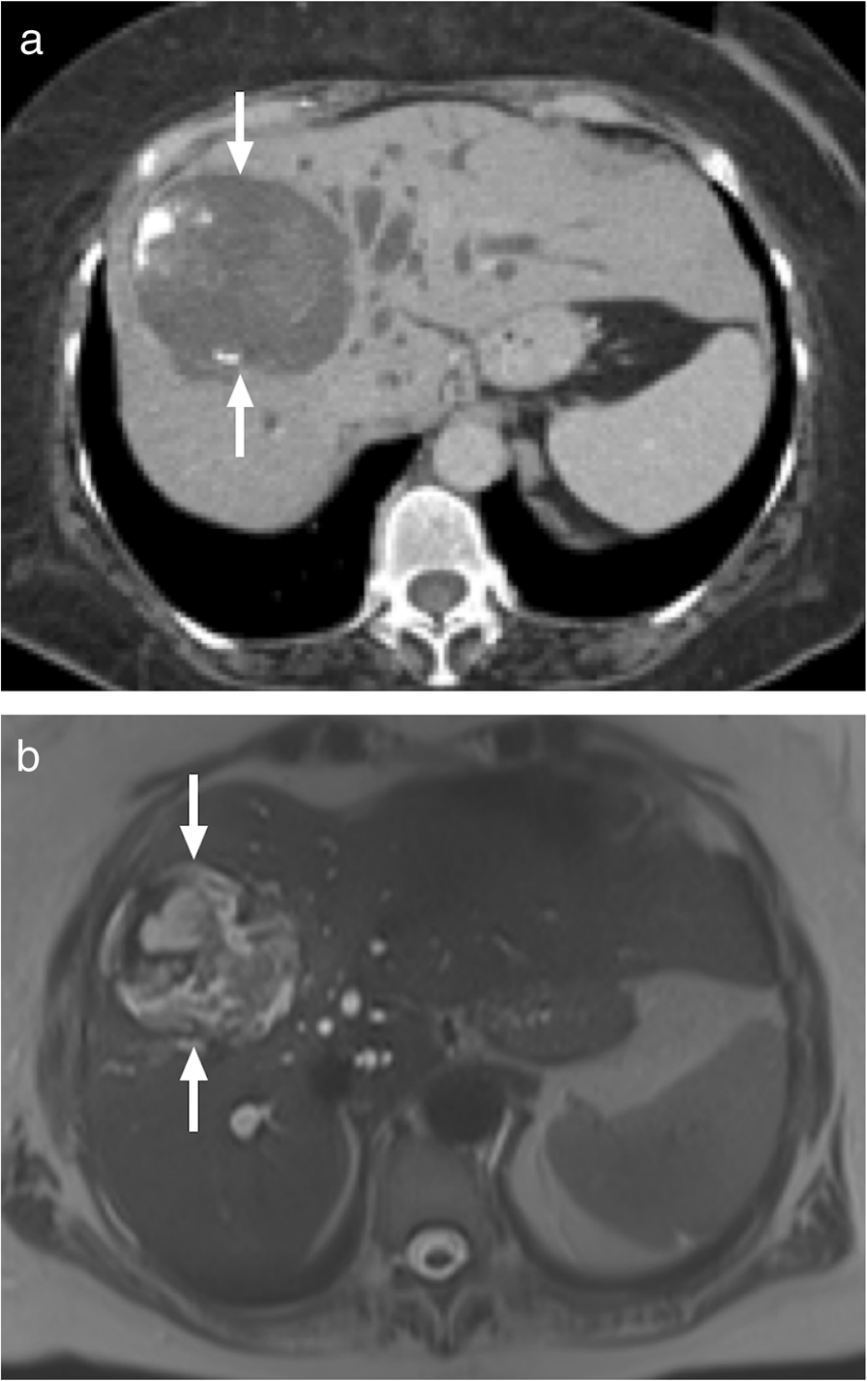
A 77-year-old male farmer with no significant medical history presented with recently onset jaundice and right upper quadrant pain. a Axial plane postcontrast abdominal CT demonstrates a complex looking mass with heterogenous central part and peripheral calcifications (arrows). Also note dilatation of the intrahepatic bile ducts due to the mass effect of the lesion. b Axial plane T2W MR image demonstrates the cystic nature of the mass (arrows) with internal septations which may suggest detached eccinochocal membrane. There was no evidence of internal contrast enhancement on postcontrast dynamic T1W images (not shown). Percutaneous drainage confirmed hydatid cyst
